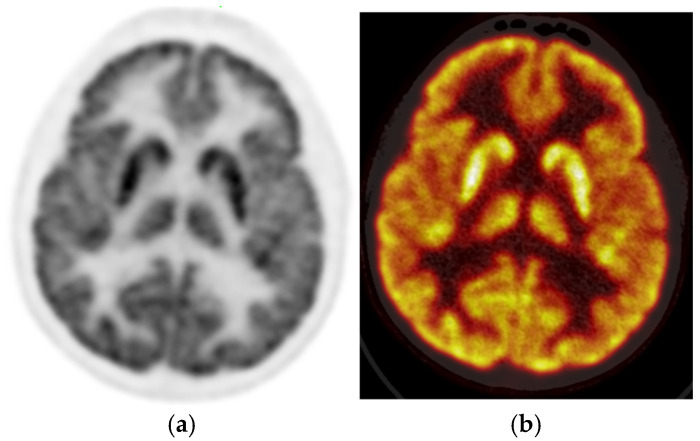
Brain 18F-FDG PET, (a) (sagittal grey scale) and (b) (sagittal rainbow color) in a 16-year-old male with no definite cortical abnormality. The uptake of the thalami is also symmetric.
